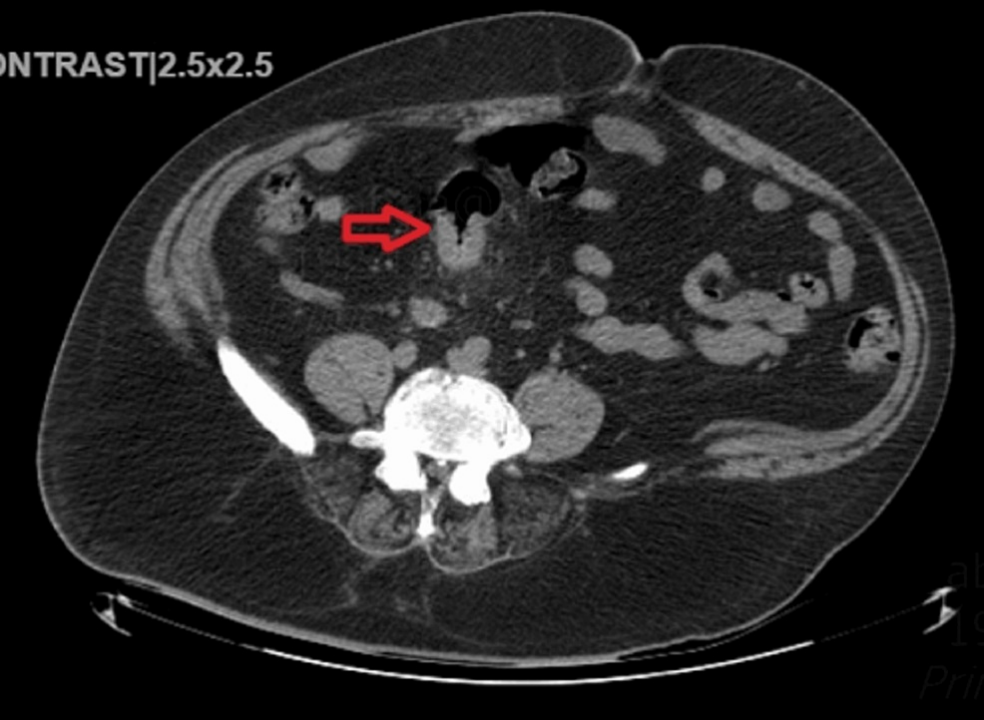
CT abdomen revealed circumferential wall thickening of the sigmoid colon (red arrow).
